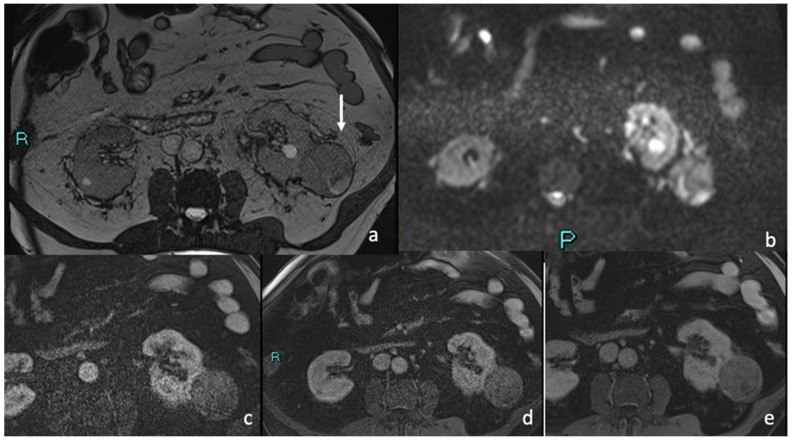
Axial T2-weighted (a) right cortical mesorenal mass with well-defined margins hypointense on T2-weighted image ((a), as shown by the arrow). (b) When using diffusion-weighted spin-echo imaging (DWI), the lesion show restricted diffusion, allowing for a correct differential diagnosis from a hemorrhagic cyst. In the postcontrast image (arterial phase (c), venous phase (d), and delay phase (e)), there is minimal, faint, irregular, spot-like enhancing foci within the lesion, with no considerable washout.
